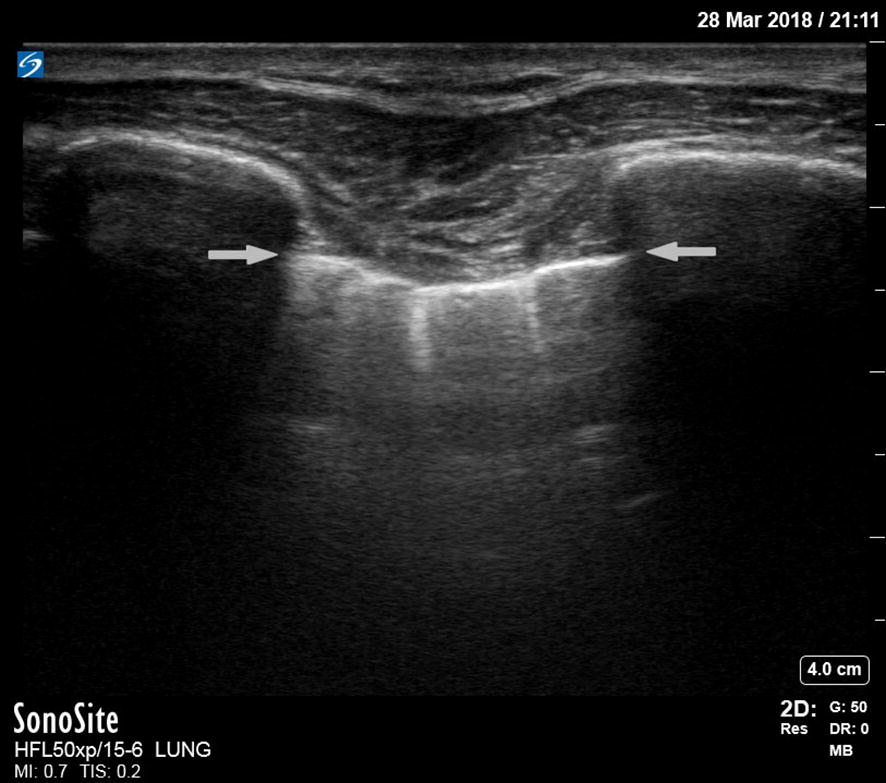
Normal lung. A normal lung ultrasound image acquired with a 15–6 MHz linear transducer. The ribs are visible with their anechoic shadows on both sides of the image. The pleural line is shown in between the ribs, indicated with two horizontal arrows. Emanating down from the pleural line are comet tails. B-lines (not visible here) also start at the pleural line, but extend all the way down to edge of the image
